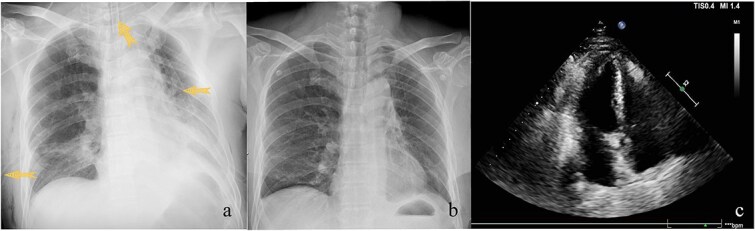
Postoperative imaging. (a) Chest radiograph obtained on postoperative day 1 demonstrating bilateral thoracic drainage tubes and an endotracheal tube (arrows). (b) Follow-up chest radiograph at discharge showing no evidence of pneumothorax or pleural effusion. (c) Postoperative transthoracic echocardiography confirming absence of residual left atrial mass.
